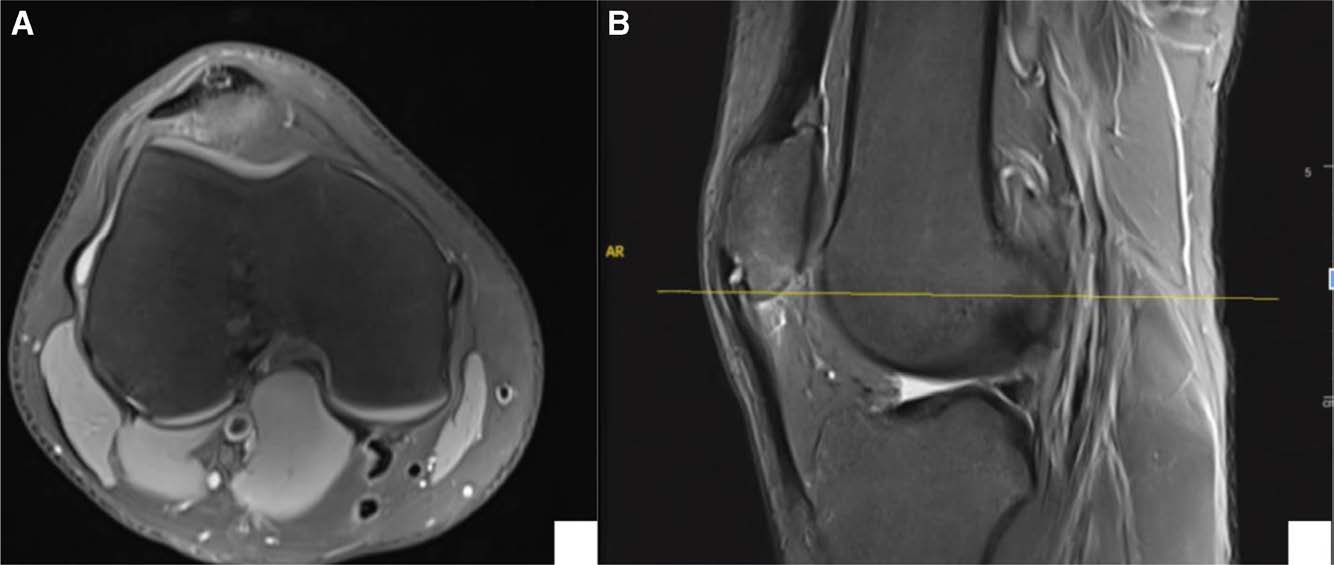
Early MRI of the knee demonstrating a Sinding-Larsen–Johansson lesion at the inferior pole of the patella. (A) Axial T2-weighted image showing focal bone marrow edema and irregularity at the patellar attachment of the patellar tendon. (B) Sagittal T2-weighted image highlighting the cortical irregularity, subchondral edema, and associated soft tissue changes consistent with osteochondrosis. MRI = magnetic resonance imaging.
